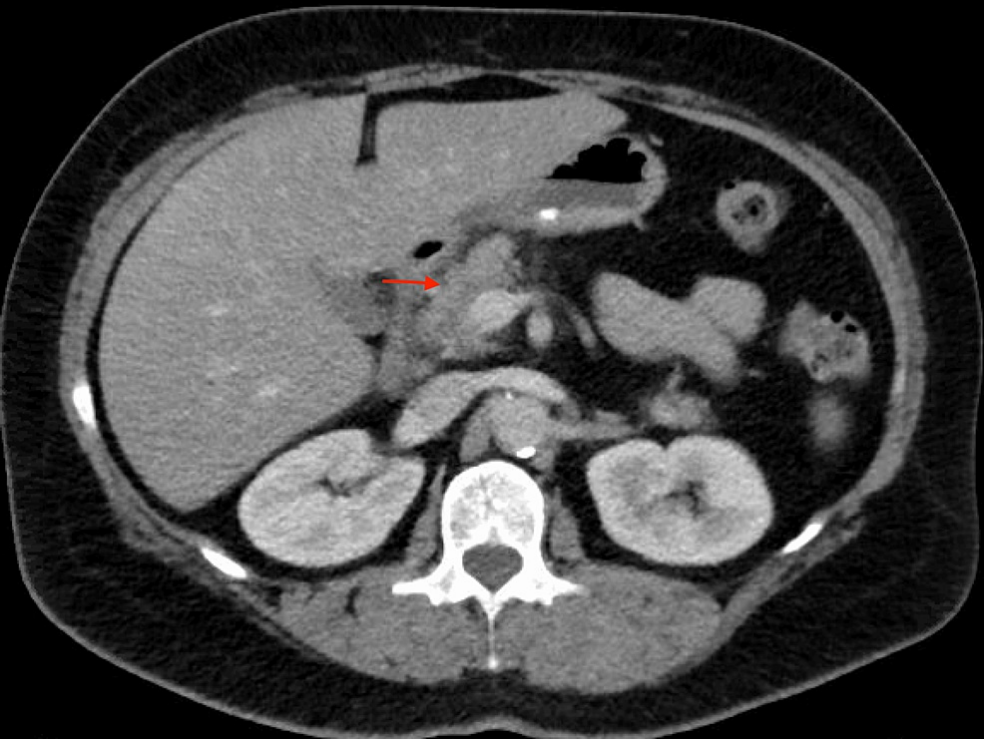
CT of the abdomen of a 51-year-old female with hypertriglyceridemia-induced pancreatitis showing ill-definition at the surface of the pancreatic head that is concerning for acute pancreatitis (red arrow)
